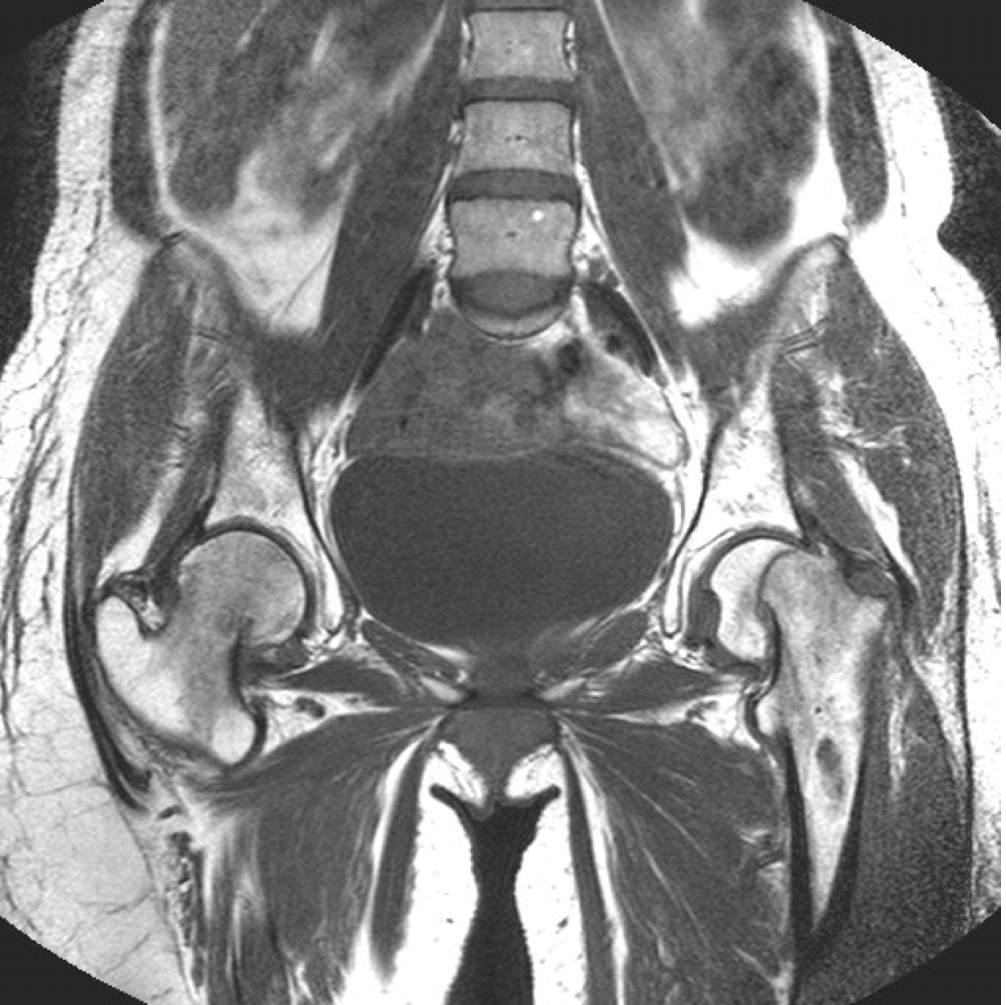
T1 weighted coronal MRI scan of the pelvis post partum.
